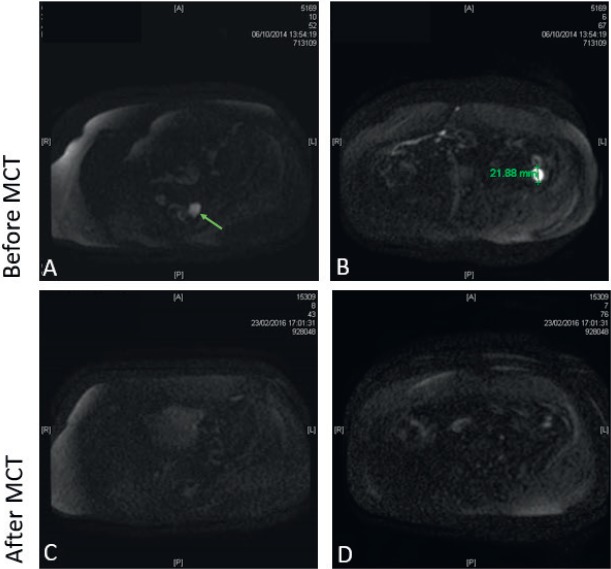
Pelvic magnetic resonance imaging using diffusion-weighted image. (A) Solid tumour lesion (arrow) of 2 cm pararectal space left rear; (B) a 2.2 cm tumour lesion in left paracolic pit. Both with enhancement in the diffusion restriction sequences; (C and D) after MCT, there are no signs of those lesions.
